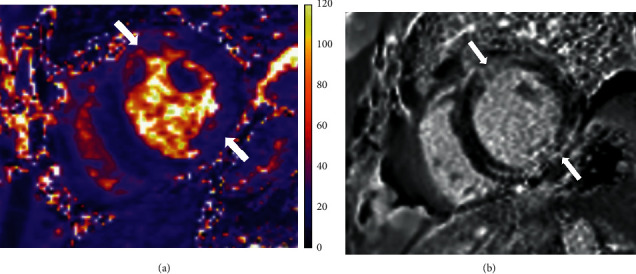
Cardiac magnetic resonance imaging of parasternal short axis. (a) T2-weighted images demonstrating extensive myocardial T2 elevation (arrow) of the left ventricle (LV) consistent with myocardial edema. (b) Extensive LV patchy, epicardial pattern of injury by late gadolinium enhancement (arrow) consistent with cellular membrane injury; there was no infarct scar seen. There was severe LV systolic dysfunction with an ejection fraction of 25%. These findings are consistent with a diagnosis of acute myocarditis.
